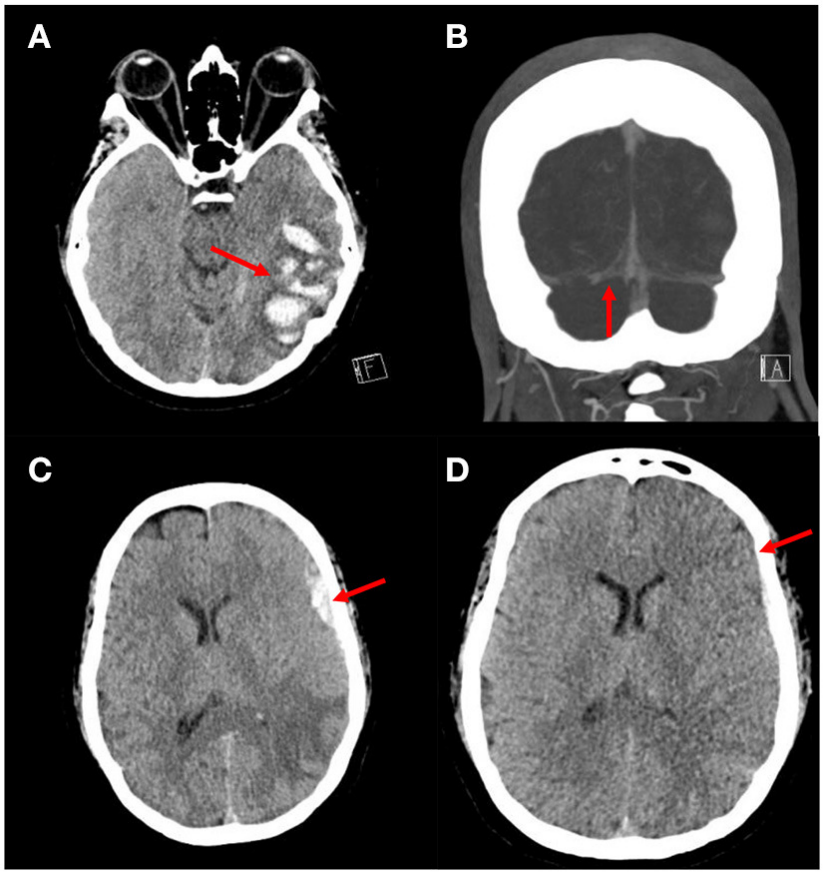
Images from patient who underwent MMA embolization followed by anticoagulation for management of aSDH with CVST. (A) Axial head CT demonstrating an ill-defined intraparenchymal hemorrhage in the left temporal lobe concerning for a venous infarct. (B) Coronal CTV showing occlusion of the right transverse sinus. (C) Axial head CT demonstrating an acute left frontal subdural hematoma. (D) Axial head CT demonstrating decreased size of the left frontal subdural hematoma.
